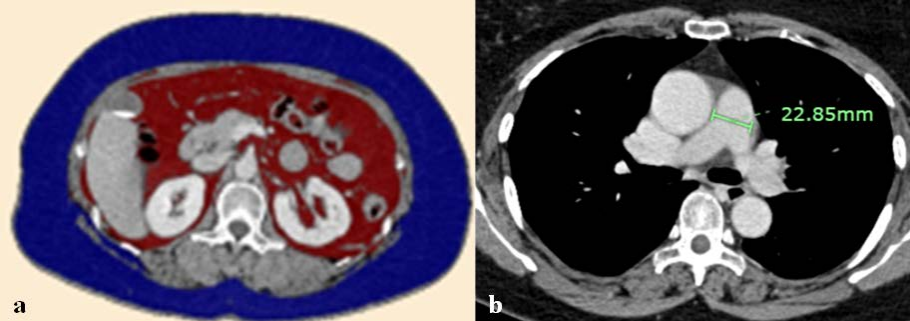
A 64-year-old woman with a BMI of 25.33 kg/m^2^ who survived after 18 months of EGFR-TKI treatment. The axial baseline post-contrast CT scan (a) at L1 level showed SAT = 275.24 cm^2^ (blue label) and VAT = 124.54 cm^2^ (red label) (b) at the level of the pulmonary artery bifurcation showed MPA size = 22.9 mm. BMI; body mass index, EGFR; epidermal growth factor receptor, TKI; tyrosine kinase inhibitor, CT; computed tomography, SAT; subcutaneous adipose tissue, cm; centimeters, VAT; visceral adipose tissue, MPA; main pulmonary artery, mm; millimeters.
